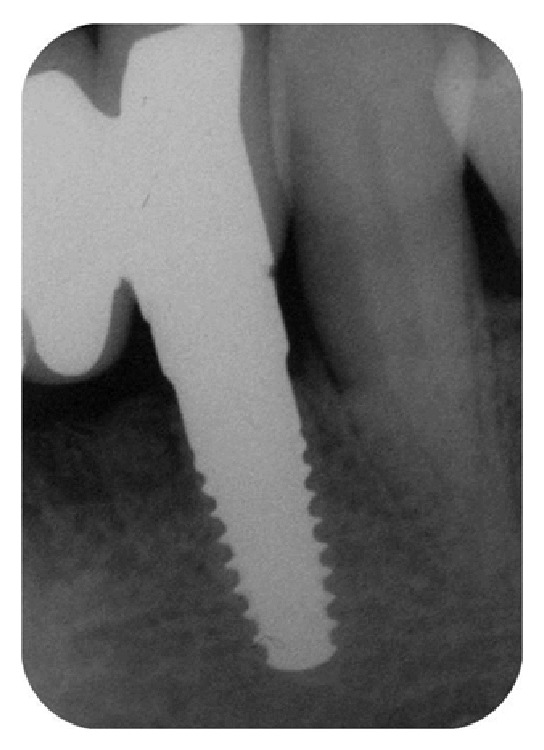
X-ray picture of the zirconia implant placed in area 3.2, six months after surgery.
